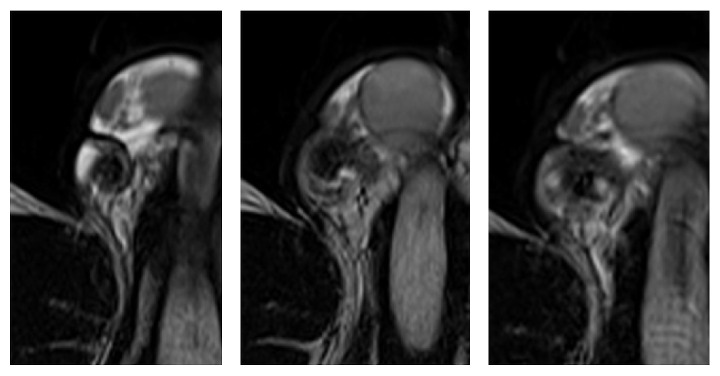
Axial T2-weighted images demonstrating right twisted spermatic cord.
